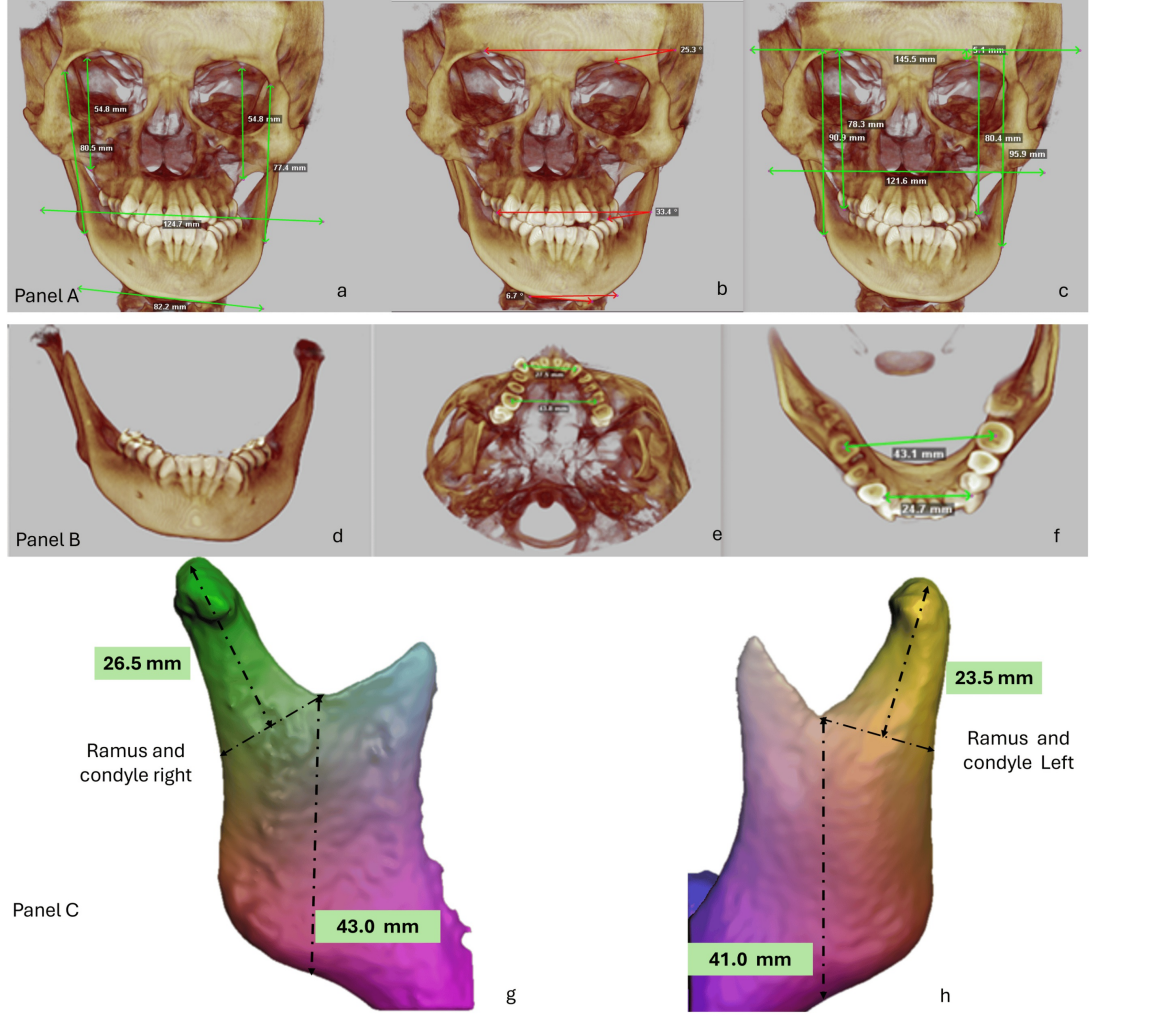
Three-dimensional reconstructions obtained from computed tomography for craniofacial and mandibular morphometric analysis. Panel A (a–c): Frontal views of the skull showing key craniofacial measurements (in millimeters), including orbital height and width, zygomatic distance, and mandibular symmetry. Panel B (d–f): Views of the mandibular complex from different angles: inferior (d), transverse (e), and oblique (f), with specific measurements such as mandibular width and interdental distances. panel C: 3D models of the right (left image) and left (right image) mandibular ramus, displaying measured heights and lengths from anatomical landmarks (values in mm). Image Credits: Dr. Gerardo Martínez-Suárez
